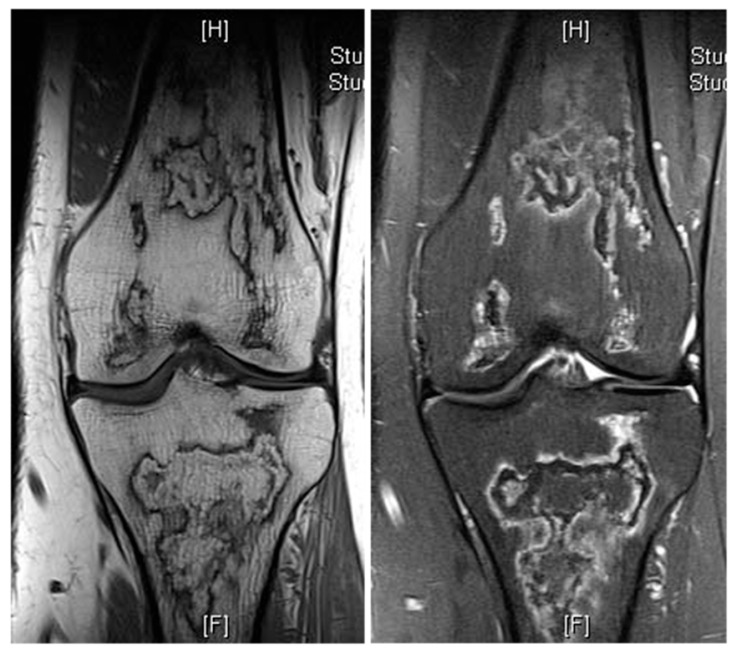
Bone infarct knee. Coronal T1W and STIR knee MRI demonstrating serpiginous, peripheral low signal intensity with central fat intensity signal (high T1 and low STIR).
